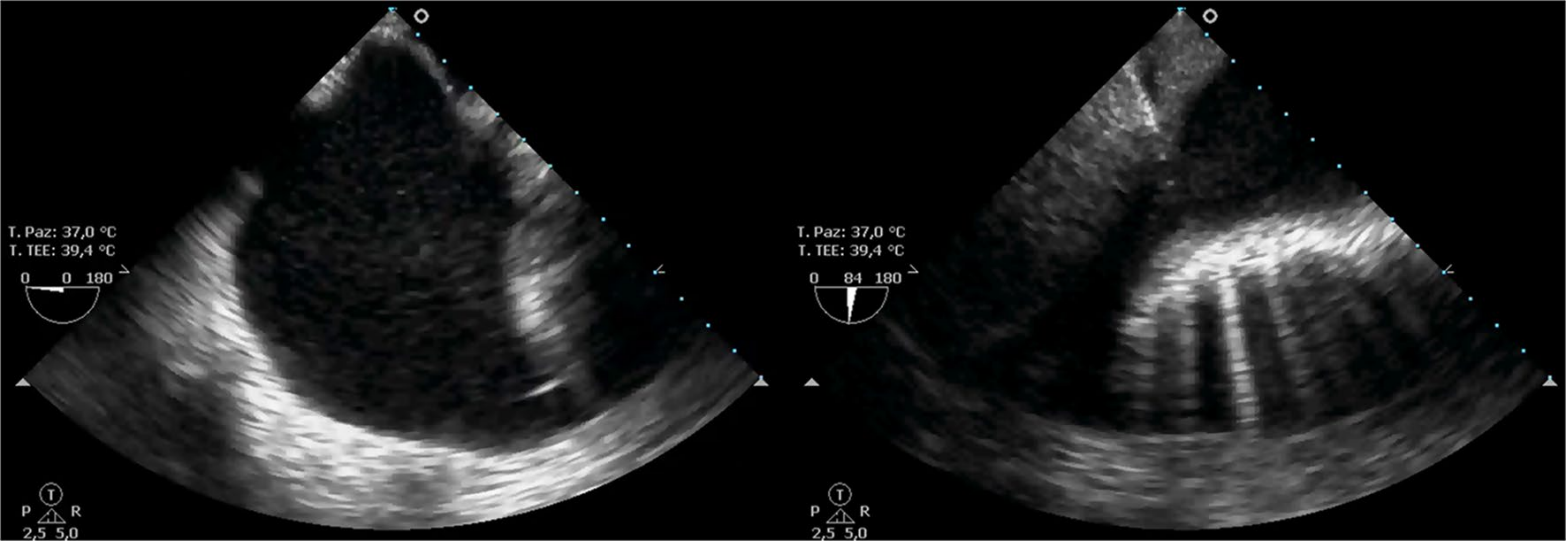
Pleural effusion in the chest cavity, as seen by trans-esophageal lung ultrasound (TELU) at 0° (*left image*) and 84° (*right image*). The effusion looks anechoic, while the lung parenchyma appears to be compressed, with some B-lines
